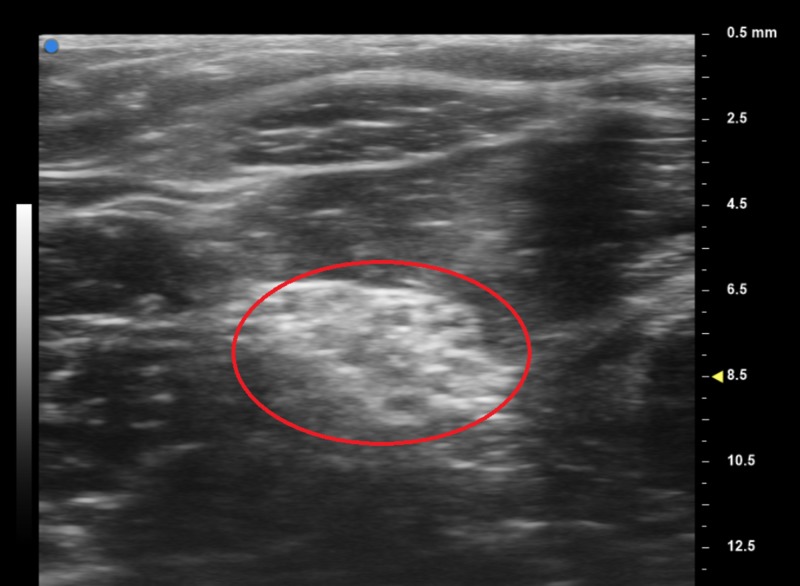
Ultra-high-frequency ultrasound (UHFUS) normal median nerve left forearm The red circle surrounds the left median nerve
